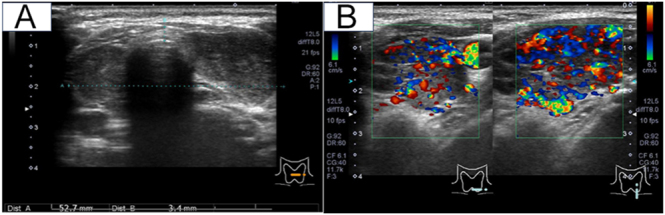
Ultrasonographic findings of the thyroid gland in the patient with Graves’ disease. (A) B-mode imaging shows diffuse goiter with a heterogeneous internal echotexture. (B) Color Doppler imaging reveals markedly increased parenchymal blood flow, a finding consistent with active Graves’ disease.
